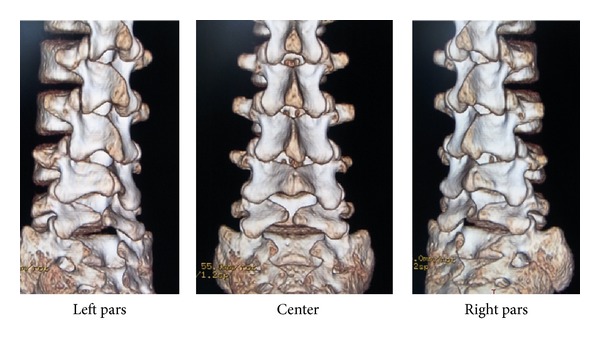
3D-CT of Case 3. Note the concomitant SBO.
